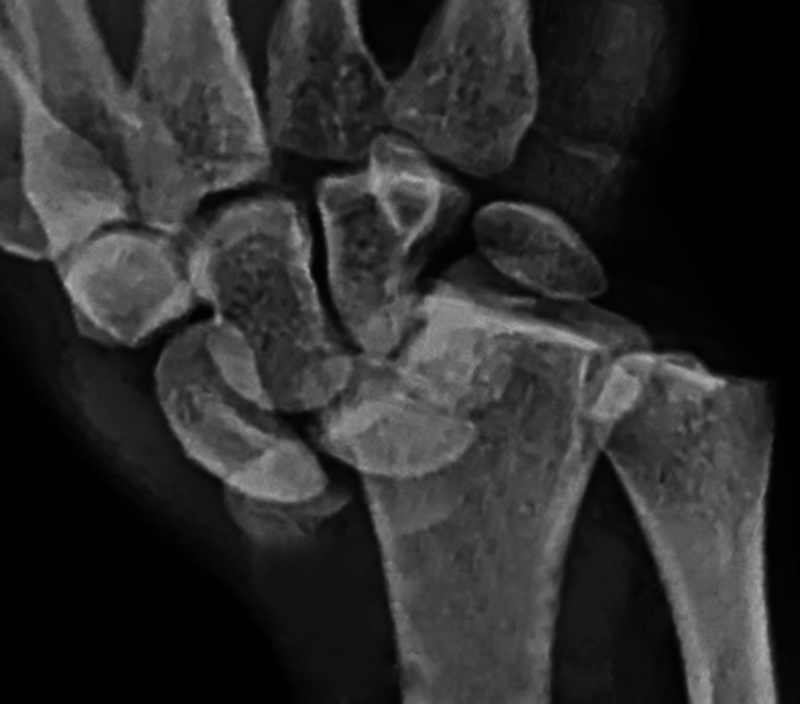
Wrist radiographic preoperative aspect – radiocarpal fractures dislocation.
